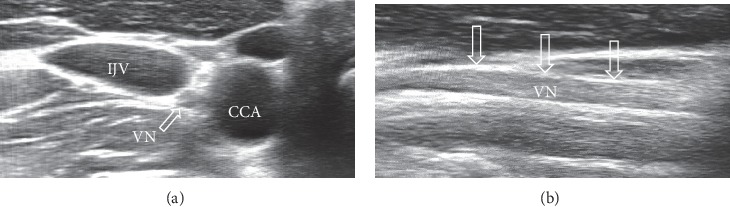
Ultrasonography scan of the vagus nerves in a healthy control subject. (a) Vagus nerve marked with an arrow in an axial image of the neurovascular bundle. (b) Vagus nerve marked with arrows in a sagittal projection. IJV, internal jugular vein; CCA, common carotid artery.
